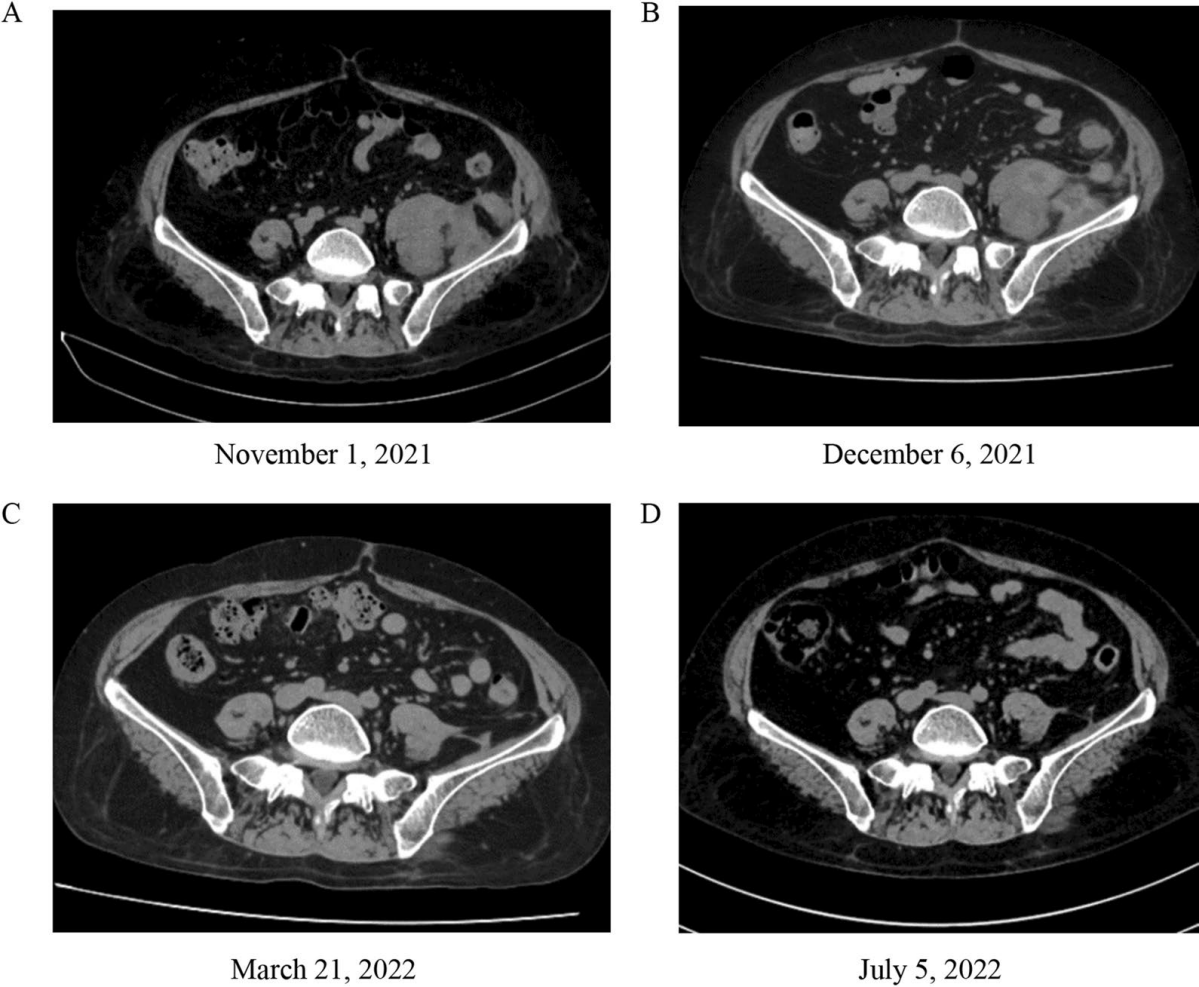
Comparison of the abdominal CT results before and after treatment. Serial CT scan images showed progressive resolution of the lesion in the left middle and lower abdomen over the course of 2 months on antimicrobial therapy: A prior to treatment; B 1 month after discharge; C 4 months after discharge; D 8 months after discharge
